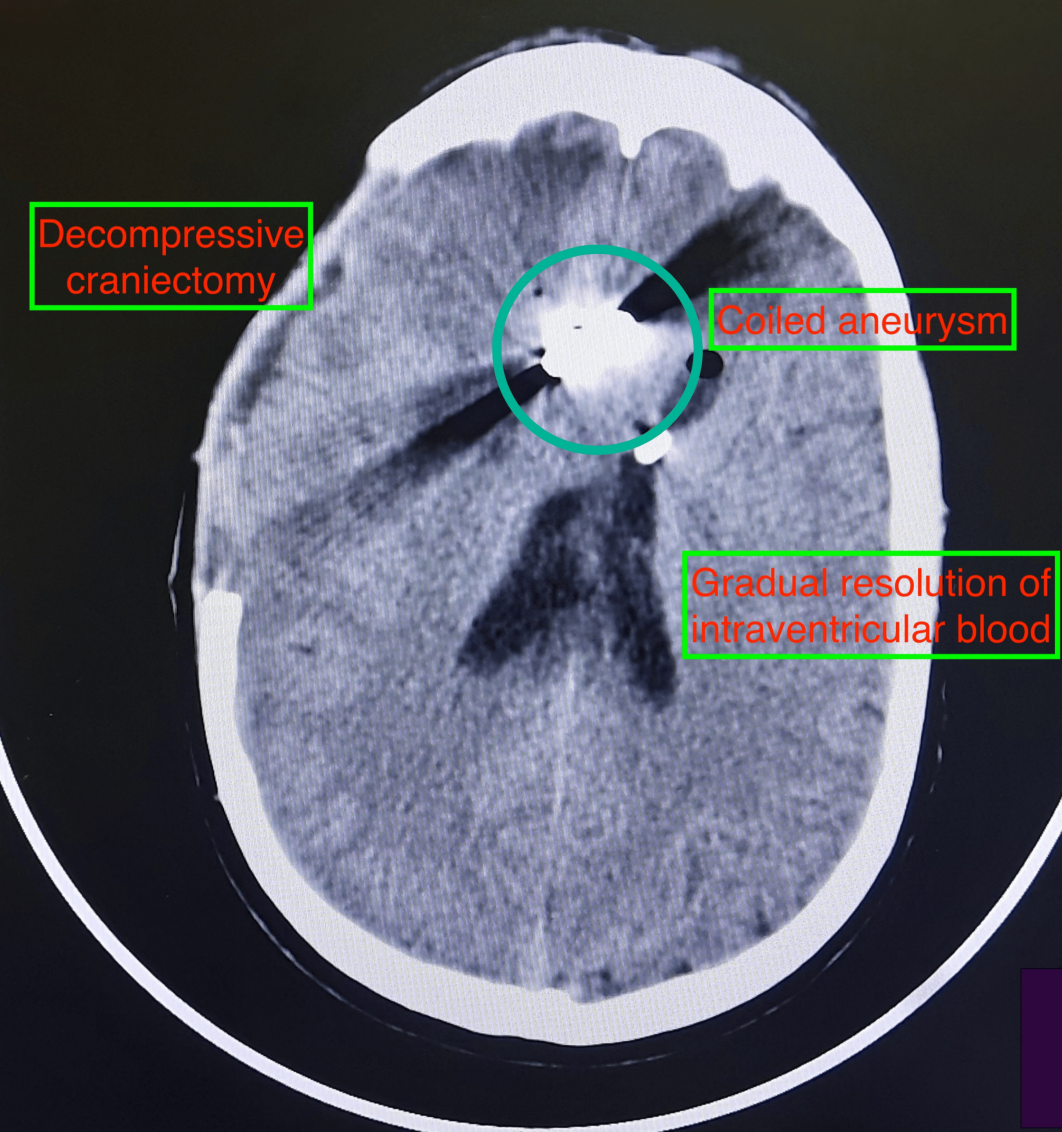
Non-contrast computed tomography of the brain showing coiled aneurysm, decompressive craniotomy, and gradual resolution of intraventricular blood.
